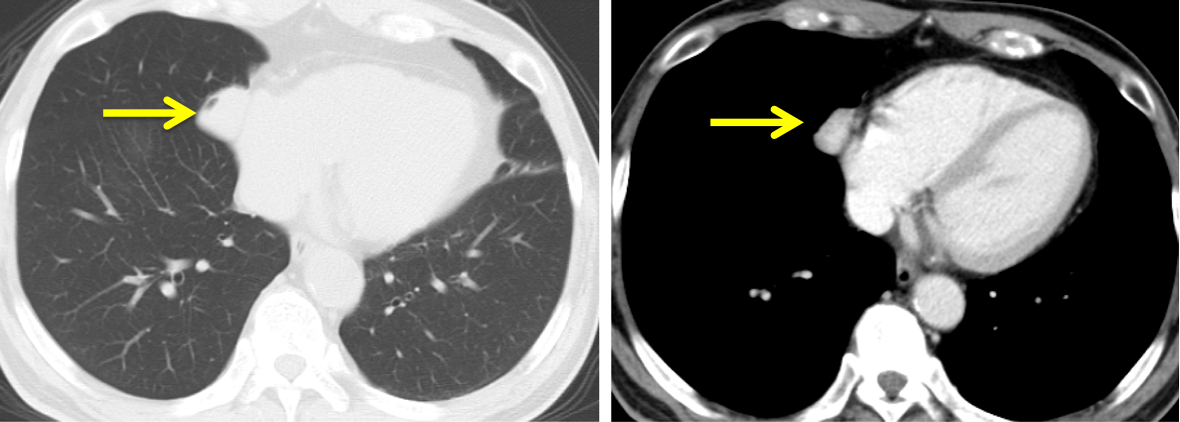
Chest computed tomography (CT) revealed the presence of a well-demarcated solid nodule of 2.6 cm in diameter that was enhanced on contrast-enhanced CT, in the cardio-phrenic angle
